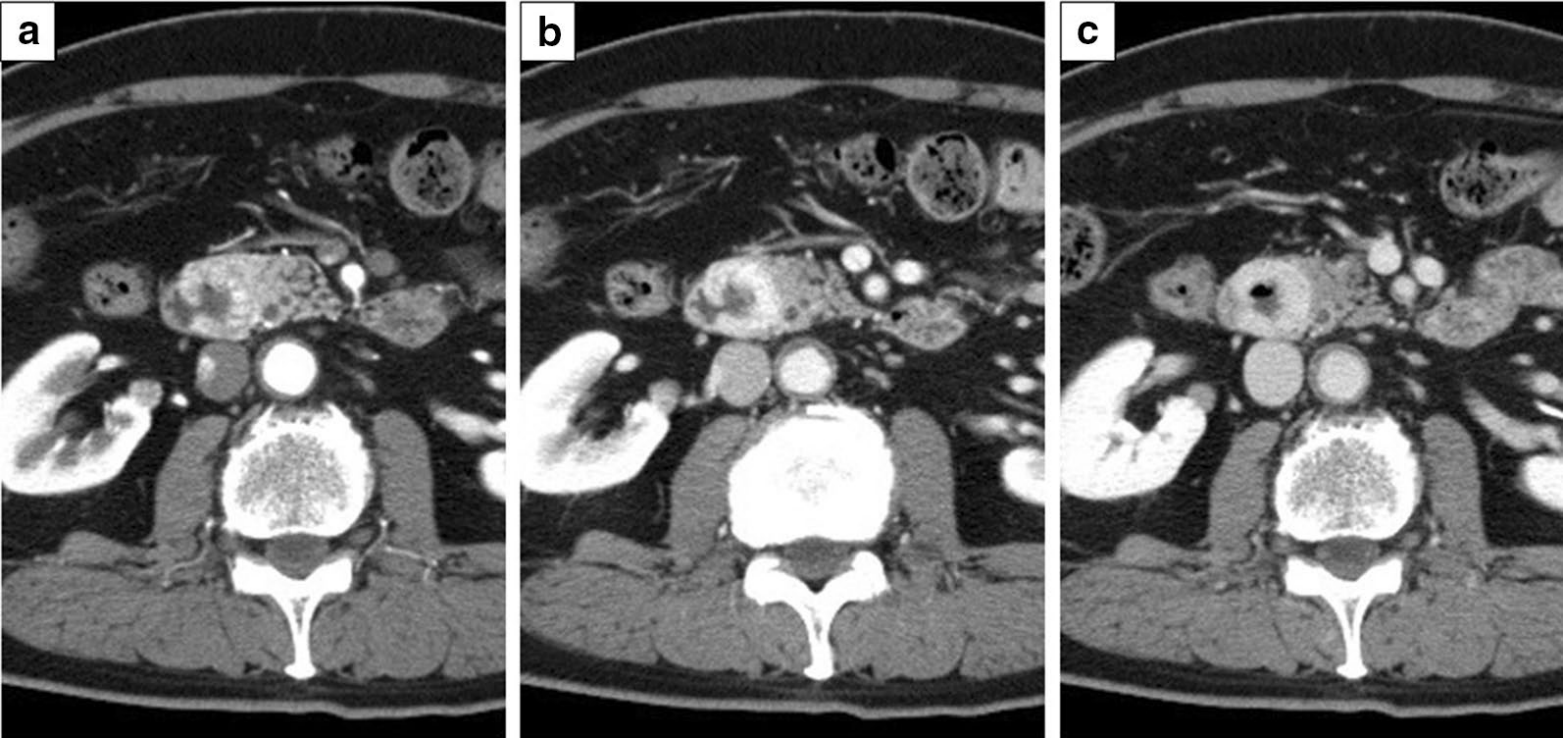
Dynamic computed tomography (CT) shows a circumscribed tumor measuring 26 mm in diameter with ulceration in the second portion of the duodenum. a, b On arterial (a) and portal phase (b) of contrast-enhanced CT, the mass reveals significant enhancement. c The mass shows persistent enhancement on the delay phase
